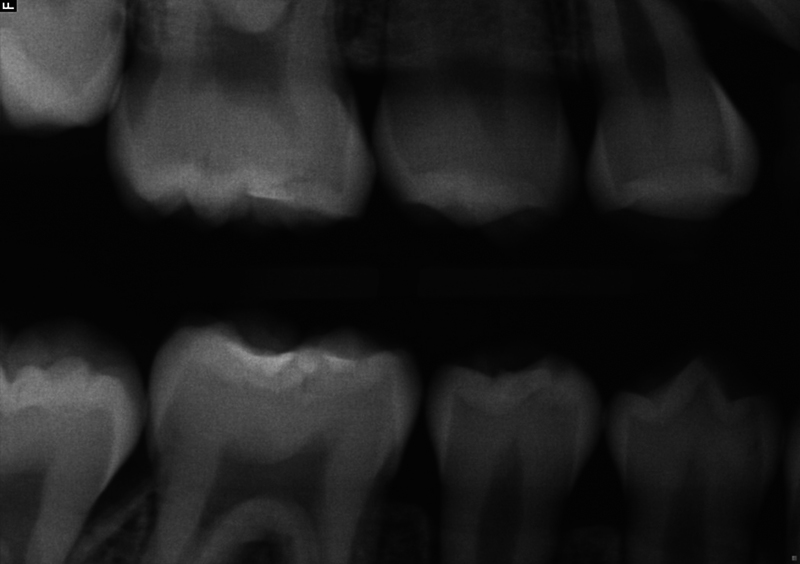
Preoperative bitewing radiograph of tooth #29 taken at the University of North Carolina at Chapel Hill Adams School of Dentistry.
